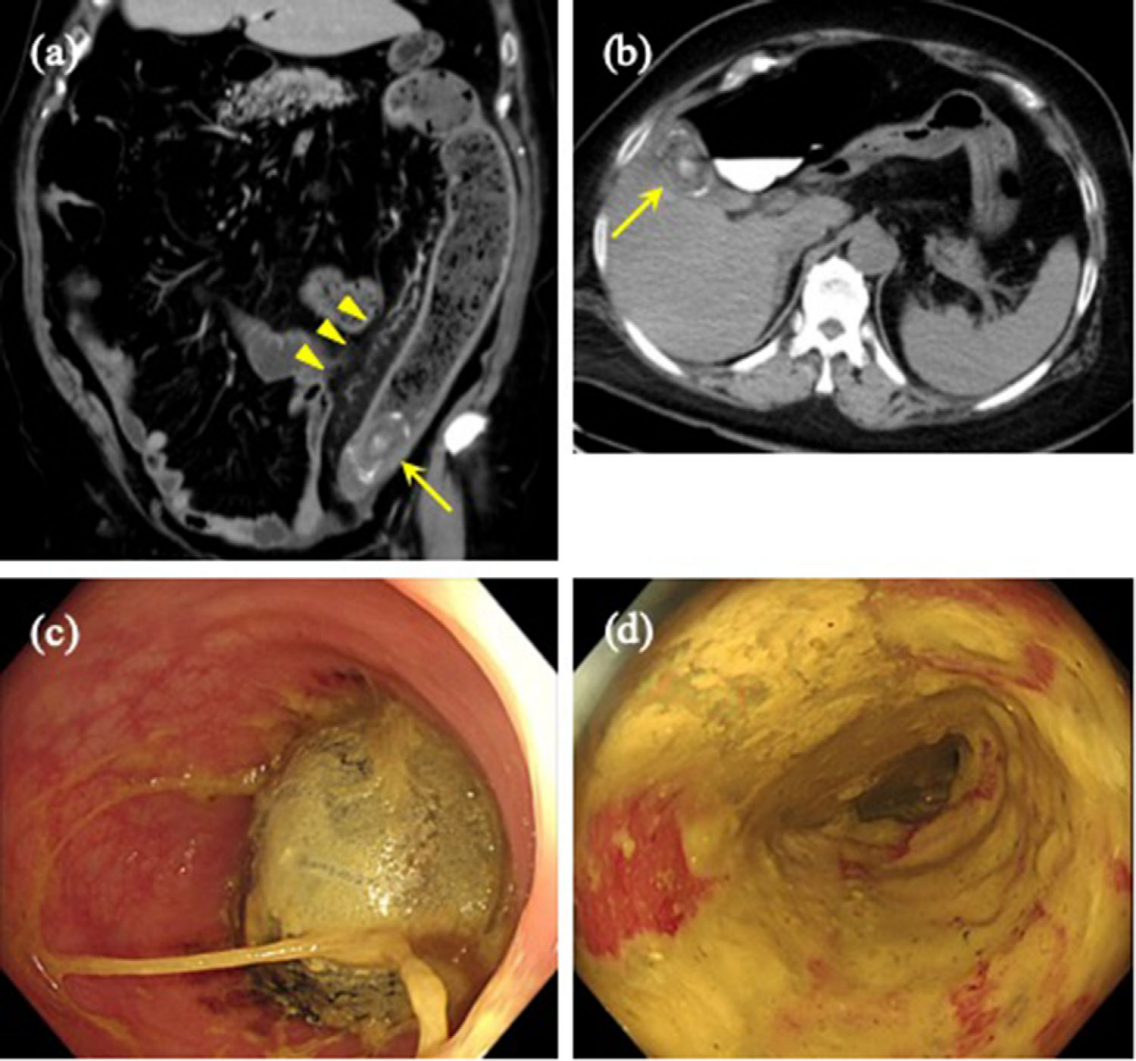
Colonic gallstone ileus. (a) Computed tomography showing a 46 × 30 mm elliptical calcification (yellow arrow) lodged in the sigmoid‐descending colon junction, and dilation of the oral colon with pericolic inflammation (yellow arrowhead). (b) A gallstone of the same measurements was confirmed in a computed tomography scan 5 years prior (yellow arrow) (c) Colonoscopy showing the gallstone impacted at the sigmoid‐descending colon junction. (d) The mucosa proximal to the obstruction appears red and edematous, compatible with obstructive colitis
